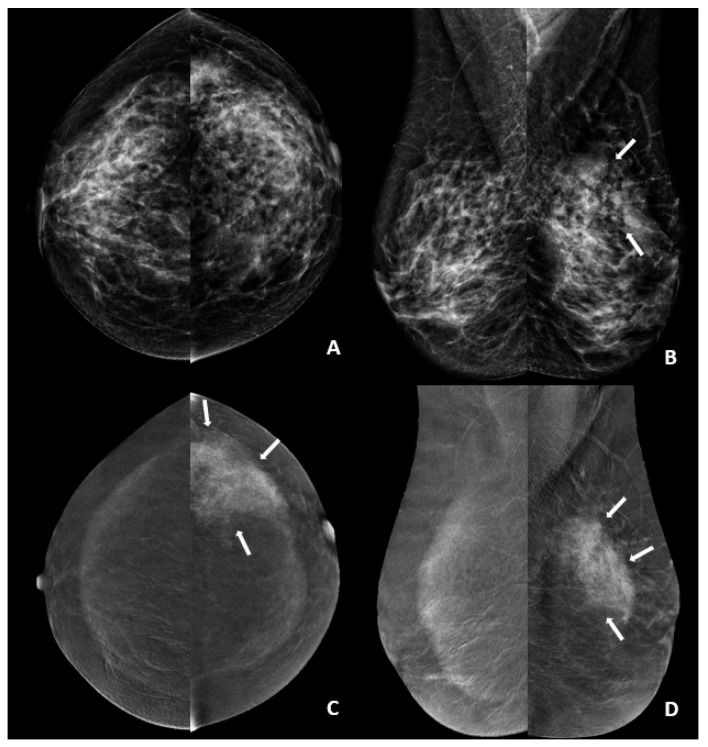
CEM images after CT examination of a 44-year-old female patient with left breast IDC diagnosis. (A,B) Low-energy CC and MLO images show an asymmetry and parenchymal distortion area (arrows) in the upper outer quadrant of the left breast. (C,D) Subtracted images show non-mass pathological contrast uptake in this area (arrows), with a maximum size of 75 mm measured on subtraction images. The scores for this patient were as follows: lesion enhancement, 4; conspicuity of the primary lesion, 4; and background parenchymal enhancement, 1.
